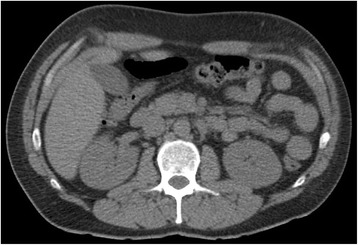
A helical computed tomography scan performed during the first emergency department visit showing no sign of urolithiasis. The kidneys are normal
